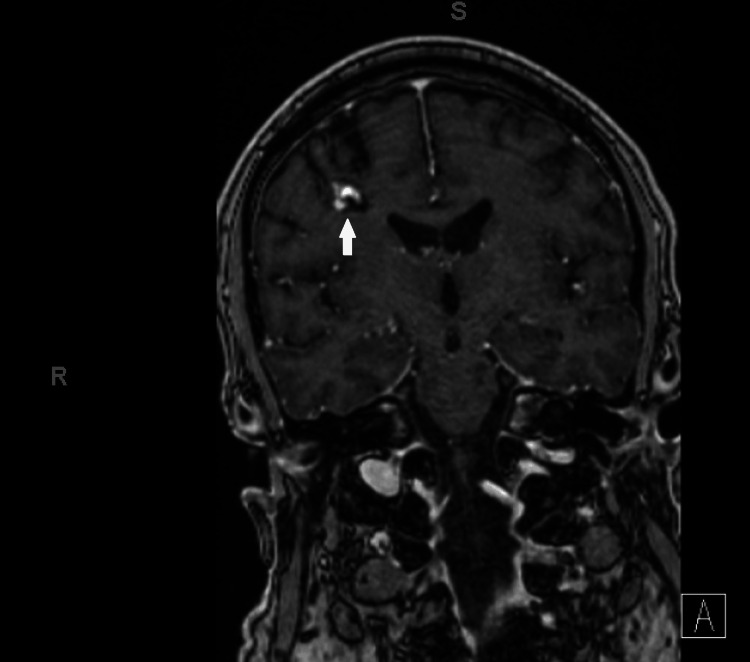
MRI brain coronal reconstruction view with IV contrast. Right frontal lobe cavernoma measuring 14mm (white arrow), no evidence of metastatic disease in the brain.
